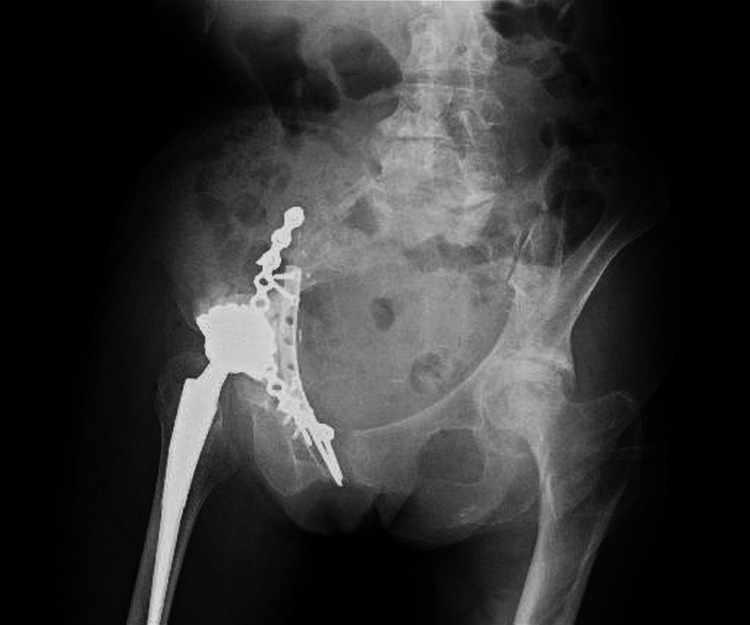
Postoperative plain radiograph of the pelvis (oblique view). The fracture was stabilized using two locking plates placed anteriorly, and the acetabular component was revised with a cemented cup.
